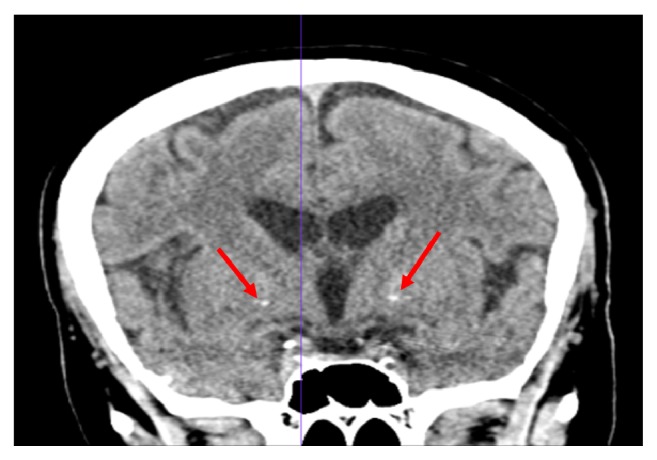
CT scan without contrast showing mild to moderate bilateral periventricular and deep white matter low attenuation along with trace bilateral basal ganglia calcification.
